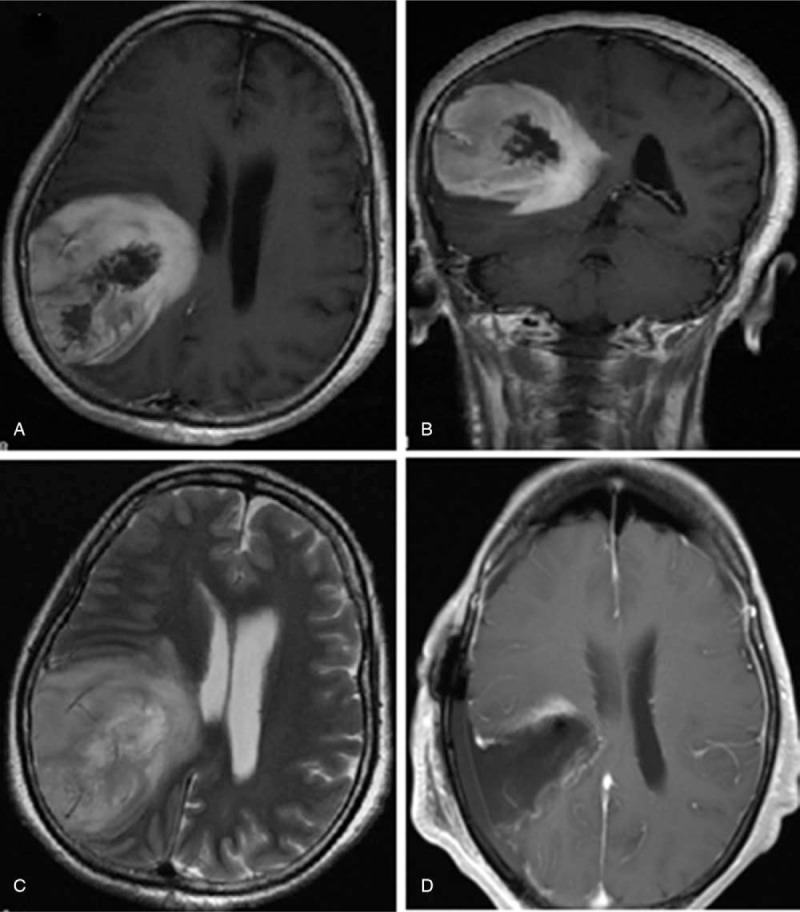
Preoperative and postoperative MRI shows a large lesion and gross total resection in a female adult with neurofibromatosis type 1 and glioblastoma. A. Axial contrast-enhance T1-weighted image shows inhomogeneous enhancement in right temporo-parietal lobe with rounded border. B. Coronal contrast-enhance T1-weighted image shows the tumor with cystic and solid component compress right ventricle. C. T2-weighted image shows a large lesion with peritumoral edema. D. Postoperative MRI demonstrates gross total resection with the tumor.
